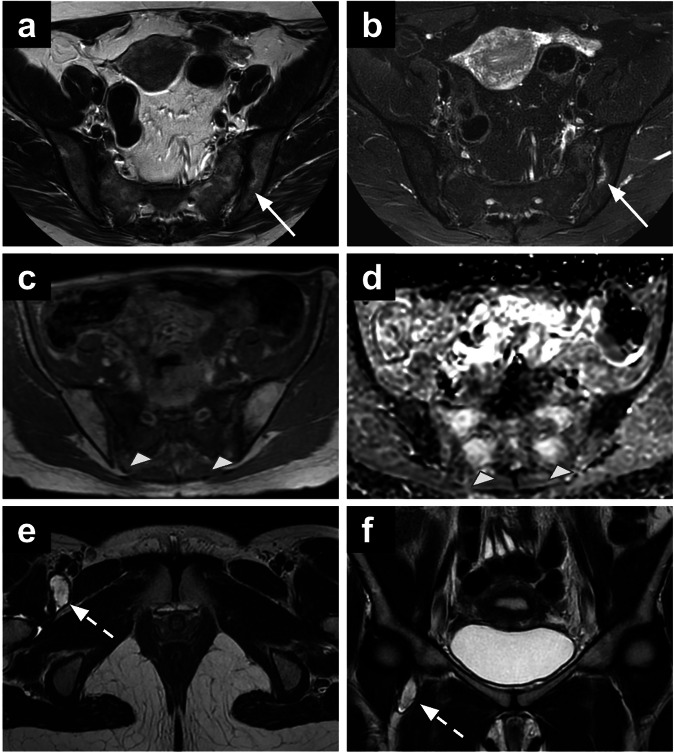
Sacroiliitis (a, b). Axial T2-WI (a) and STIR (b) images of a 50-year-old woman who underwent MRI for left ovarian lesion characterisation showing left active sacroiliitis (arrows). Pelvic insufficiency fracture (IF) (c, d). Bilateral thin obliquely oriented hypointense lines on axial T1-WI are noted in the sacrum of a 64-year-old patient on follow-up for cervical cancer treated with radiotherapy, with diffuse bone marrow oedema confirmed on ADC map, in keeping with IF. Synovial cyst (e, f). Axial (e) and coronal T2-WI (f) showing a synovial cyst arising from the right hip in a young woman with pelvic pain. STIR, short tau inversion recovery; WI, weighted images
